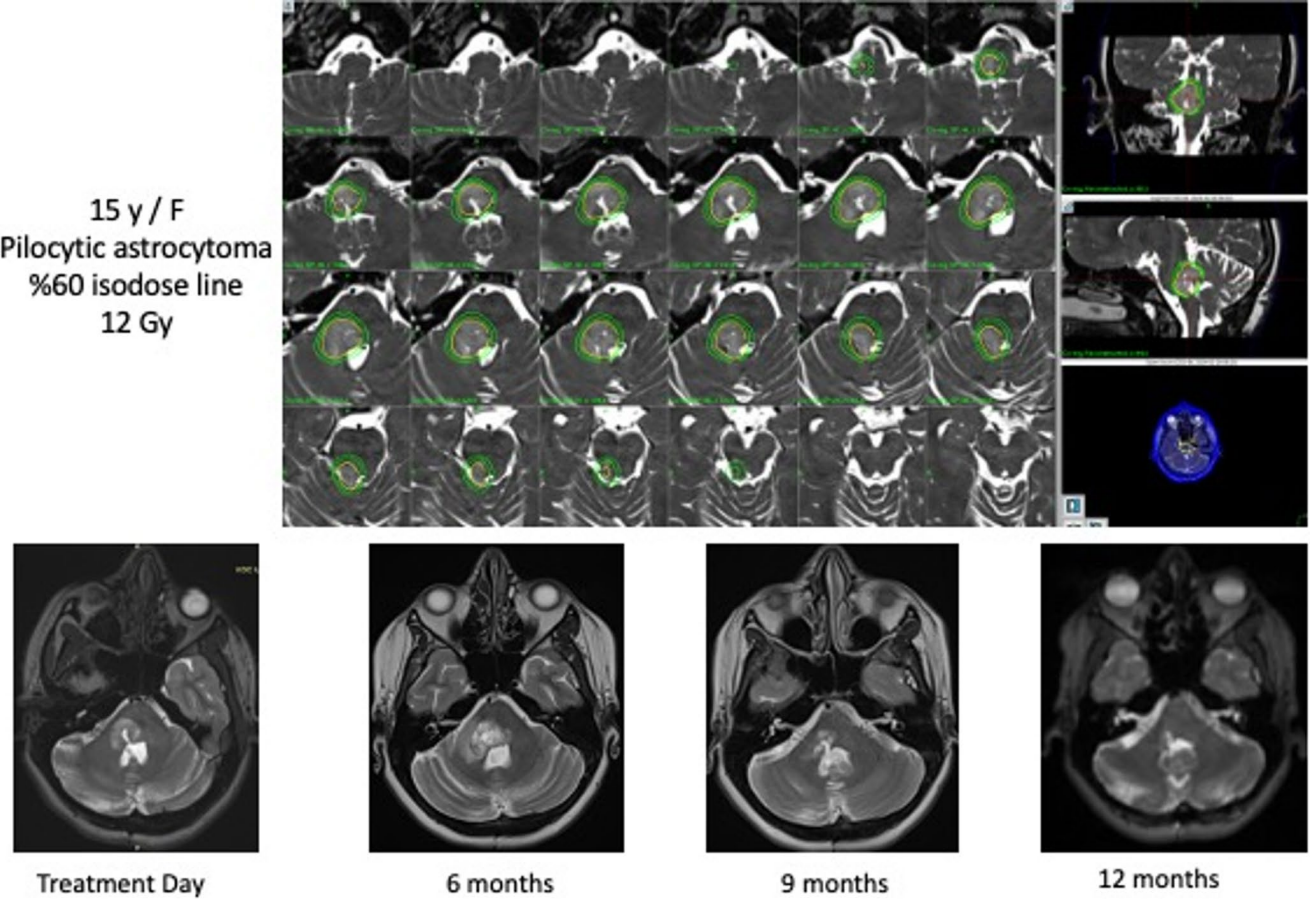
Gamma Knife radiosurgery dose planning and serial MRI follow-up of pilocytic astrocytoma
